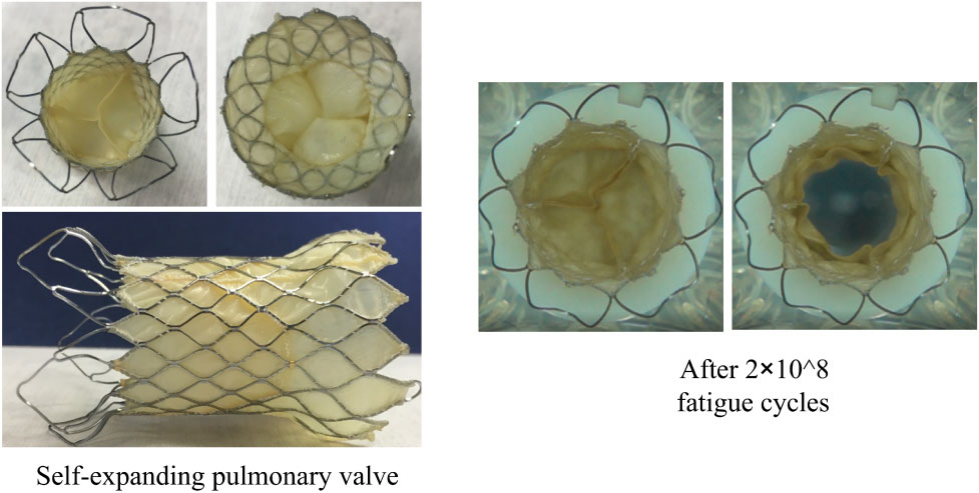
X-rays were performed and they showed no obvious calcification.
